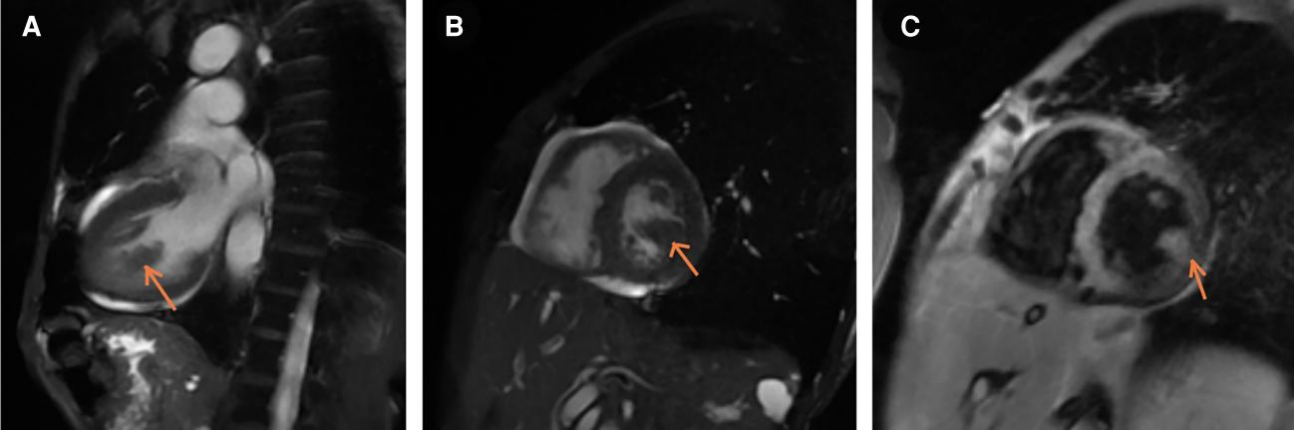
Cardiac MRI. (*A*) Long axis view: Mass from lateral wall, (*B*) Mid Short axis view at the level of papillary muscles: Mass from the lateral wall; same intensity as myocardium itself suggesting a solid mass (as compared to TTE where it had an echo-lucent core as if filled with fluid), (*C*) Gadolinium enhancement: mass enhances in a similar fashion as the lateral wall -> solid mass.
